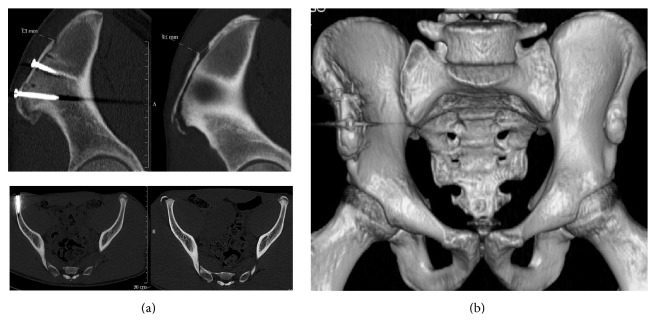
CT scan. (a) before (left) and after operation (right): reduction and internal fixation with two screws of the avulsion. (b) 3D CT scan. Good position of the fragment with two screws.
